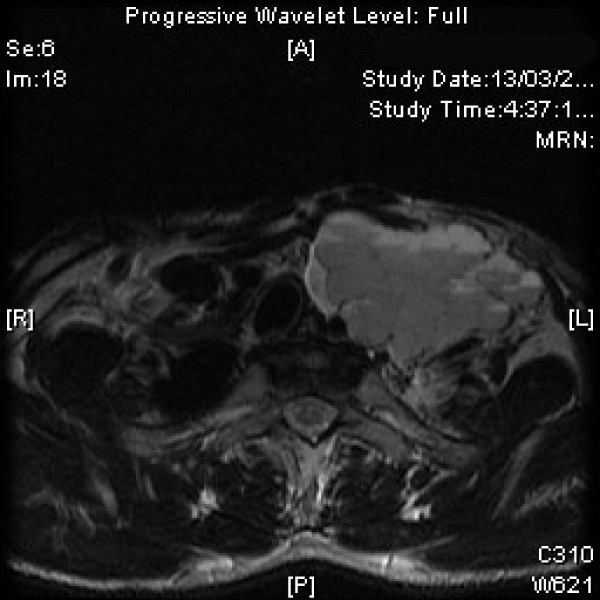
T2 W magnetic resonance image of the neck (axial section) showing hyperintense mass with fluid-fluid levels.
